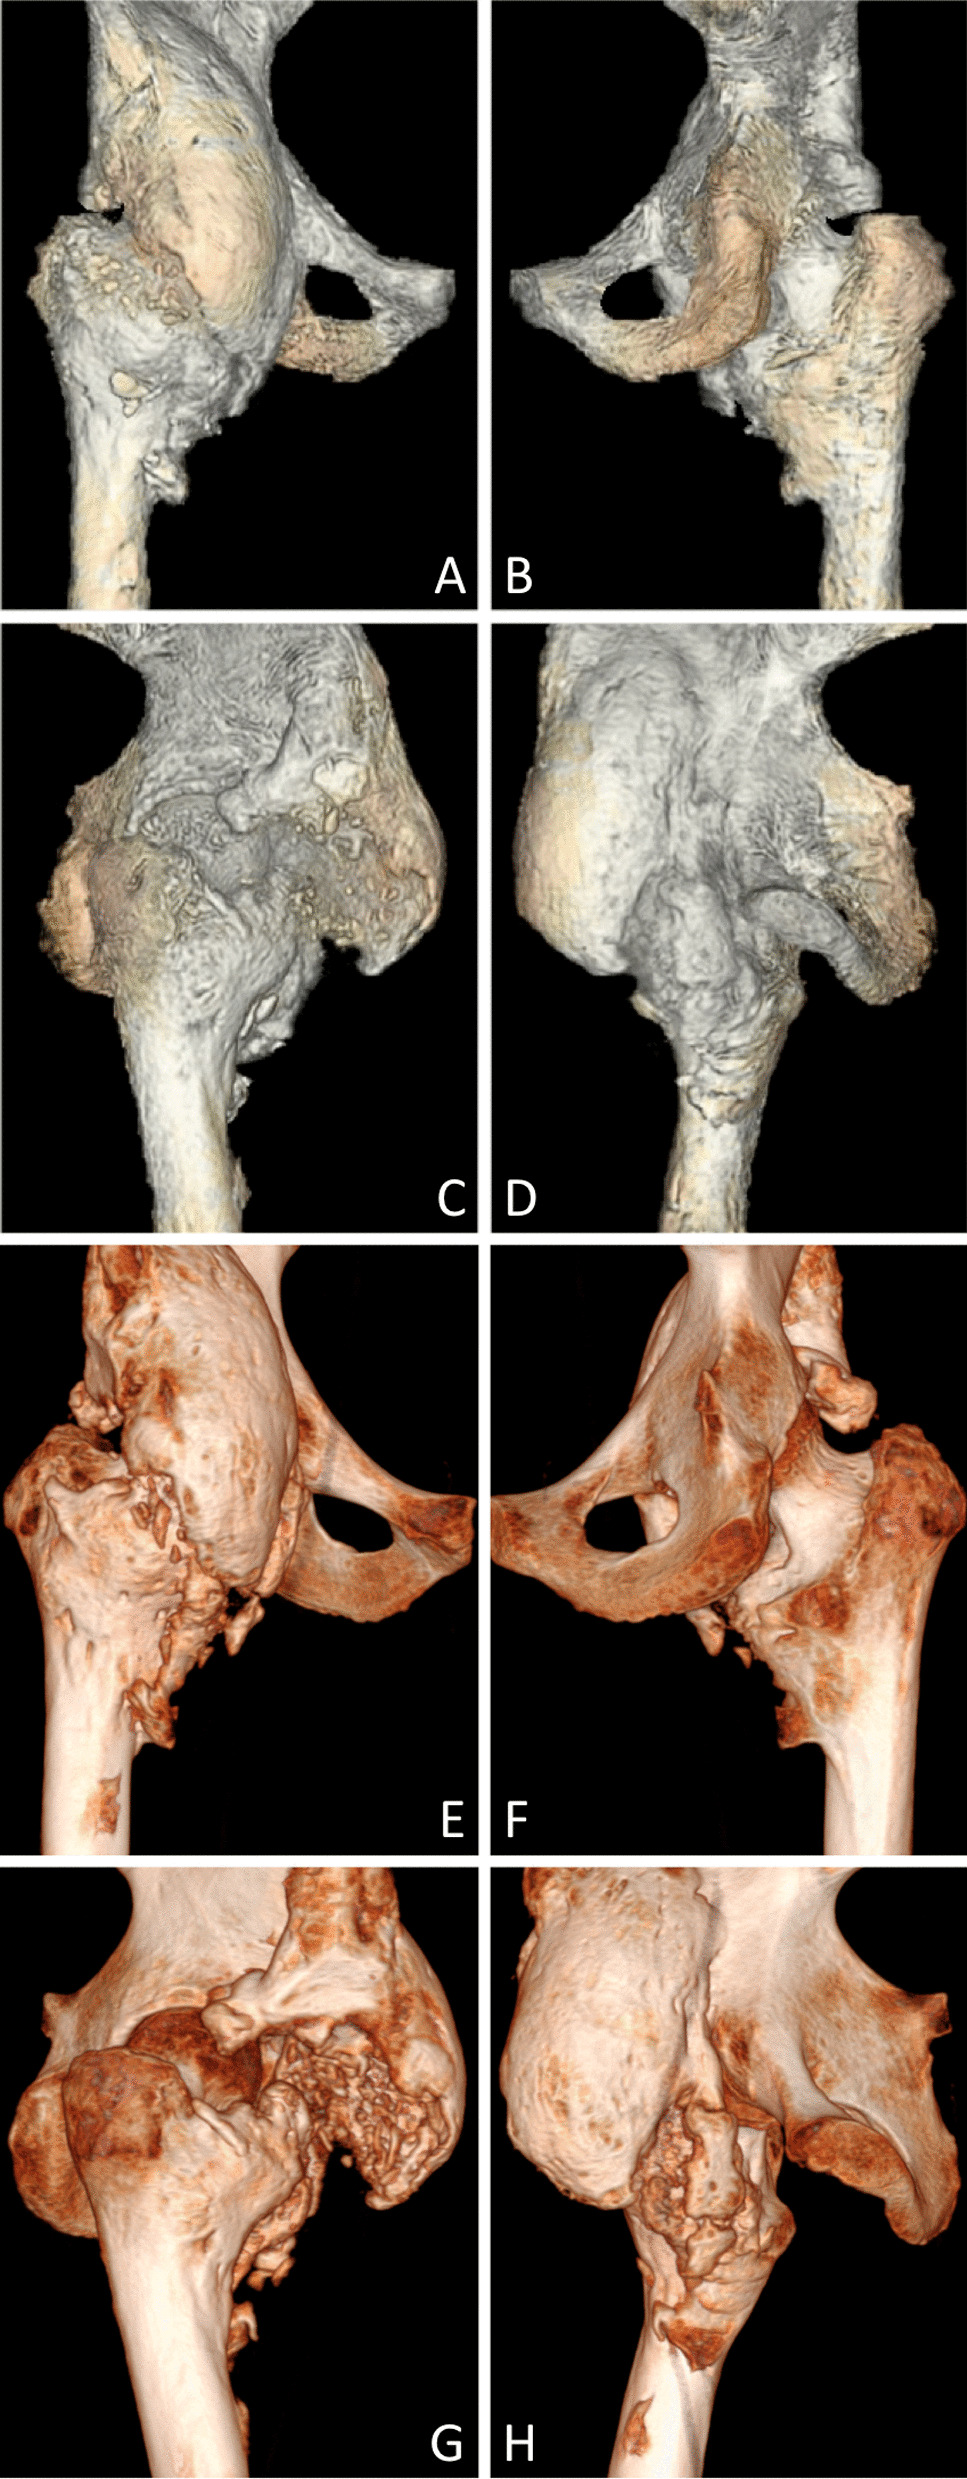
Anterior multifragmented NHO of the right hip. 3D volumic reconstructions with anterior, posterior, right and left views on ZTE sequence (A–D) and their corresponding images on CT (E–H)
